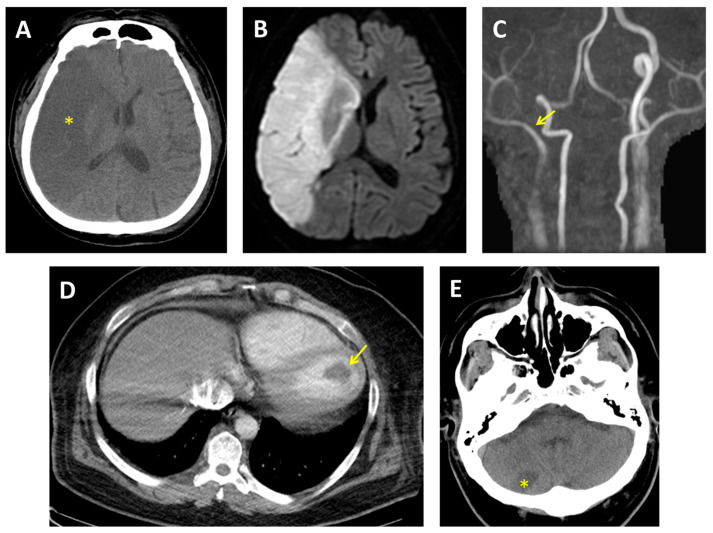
(A) Brain CT initially revealed a right middle cerebral artery territory with a large hypodensity (star). (B) Brain MRI showed hyperintensity on DWI in the same area. (C) MRA showed total occlusion of the right internal carotid artery (arrow). (D) Contrast-enhanced CT of the chest revealed a left ventricular thrombus (arrow). (E) Follow-up brain CT showed a new hypodensity in the right cerebellum (star). CT, computed tomography; DWI, diffusion-weighted imaging; MRA, magnetic resonance angiography; MRI, magnetic resonance imaging.
